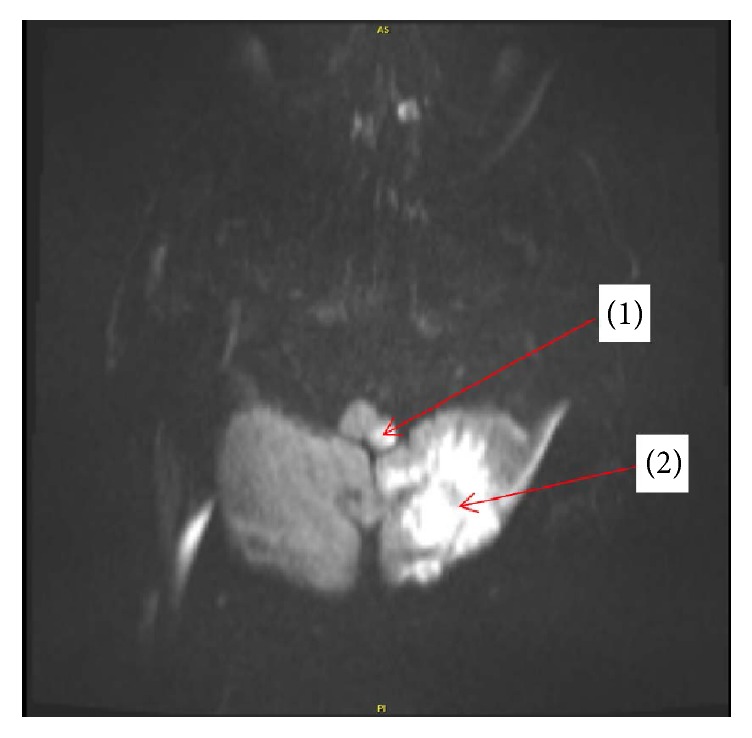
Axial diffusion weighted imaging sequence of a magnetic resonance imaging scan of the brain demonstrates hyperintensity in various brain areas. Those findings are consistent with an acute stroke in the left medullary restiform body (1) and left cerebellar hemisphere (2).
